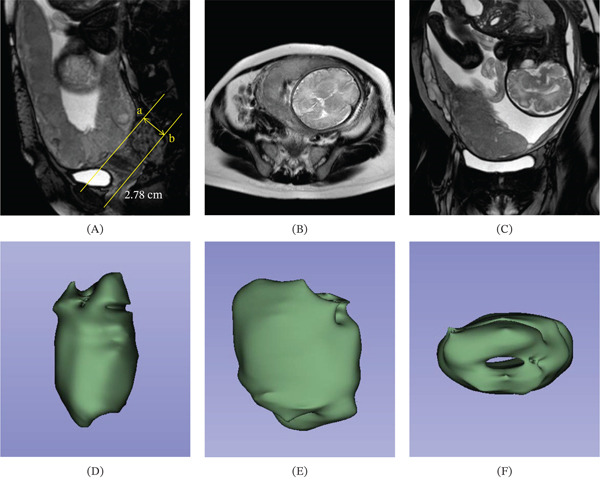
The cervical length was measured, and the cervical volume was reconstructed. (A) Cervical length was measured between lines a and b in a sagittal MRI image (2.78 cm). (B) Axial placenta image of a patient with PAS by MRI. (C) Coronal placenta image of a patient with PAS by MRI. (D) Sagittal image of the three‐dimensional cervix generated by 3D Slicer. (E) Coronal image of the three‐dimensional cervix generated by 3D Slicer. (F) Axial image of the three‐dimensional cervix generated by 3D Slicer.
